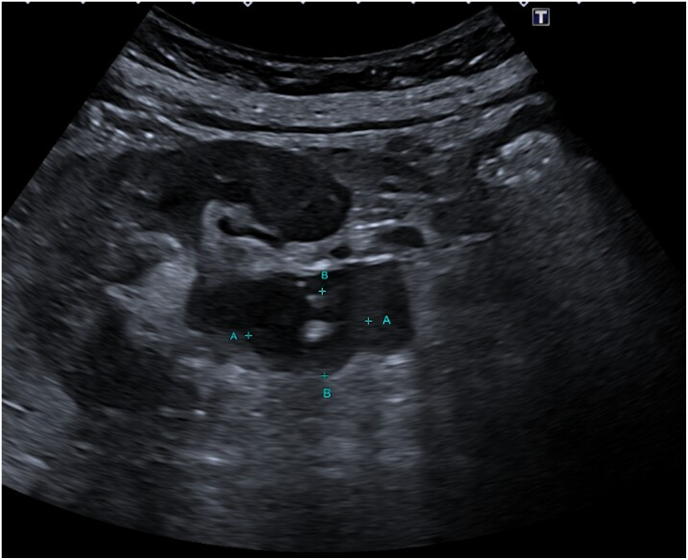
Ultrasound image of the right kidney showing a centrally located echogenic mass within the renal pelvis, surrounded by debris and without posterior acoustic shadowing or twinkle artifact. These findings are atypical for a calculus and are suggestive of a fungal ball.
